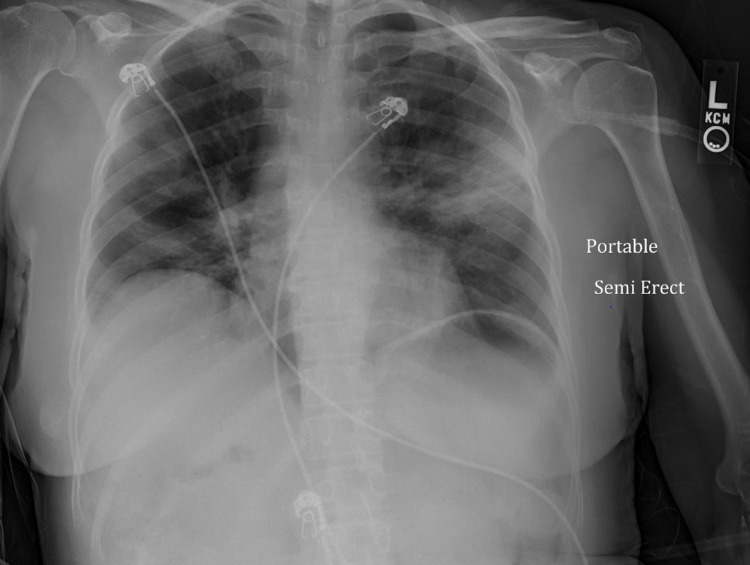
Chest radiograph revealed bilateral opacities
